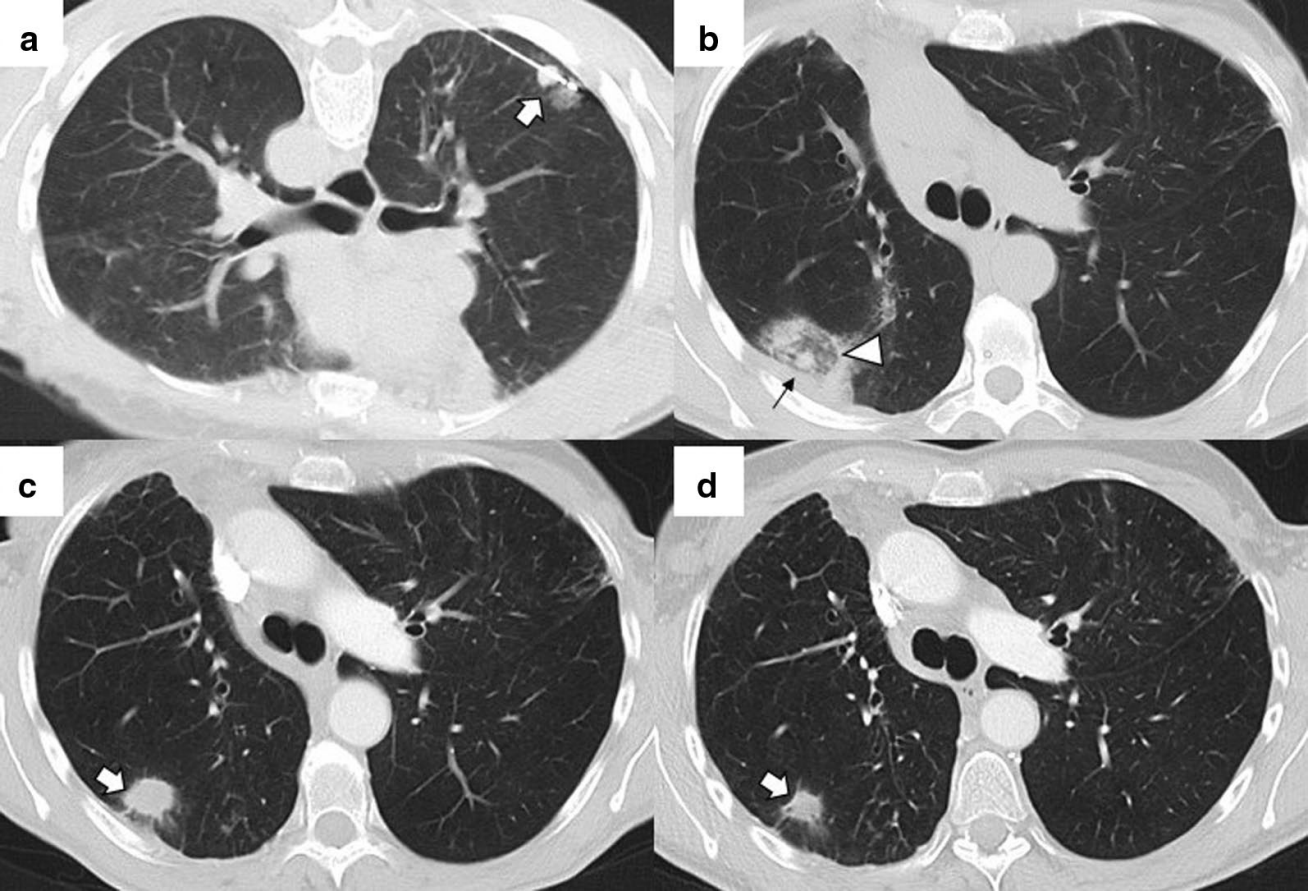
Radiological findings indicative of successful treatment immediately following image-guided percutaneous lung ablation. a CT-guided MWA with a single straight probe of a stage 1 NSCLC located in the lower right lobe. b We observed a consolidative center (black arrow) surrounded by a peripheral ground-glass halo (triangle) in the area treated in the CT scan performed immediately after completion of the procedure. The combination of these findings is indicative of successful treatment. c Follow-up CT one month after treatment. Note the increase in the size of the treated area compared to A. However, there are no irregular nodular areas nor other signs of tumor persistence. d CT 6 months after treatment The treated area has decreased in size compared to C, suggesting a successful treatment
